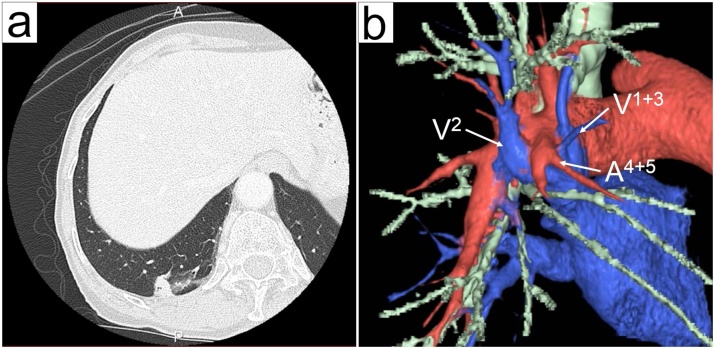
(a) Computed tomography (CT) shows a 15-mm tumor in the right lower lobe. (b) Three-dimensional multidetector CT shows that A4 + 5 is running between V2 and V1 + 3.
